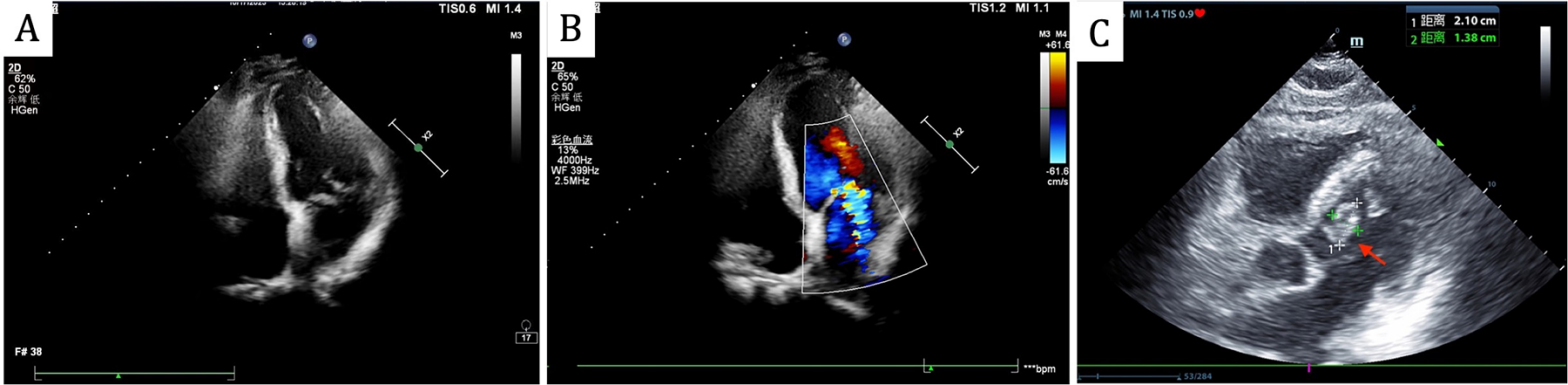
Echocardiography revealed partial “crab-like” movement of the mitral valve's anterior leaflet, and mild to moderate regurgitation on admission (A,B), the follow-up echocardiogram revealed a mitral valve mass, as indicated by the red arrow (C).
